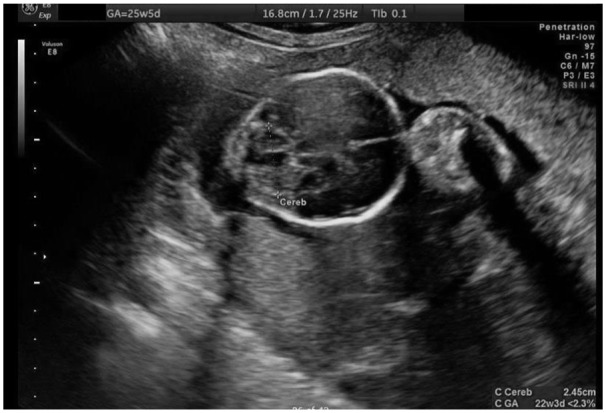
Ultrasound at 25w5d demonstrating posterior fossa with cyst/absent cerebellar vermis in Fetus C.
